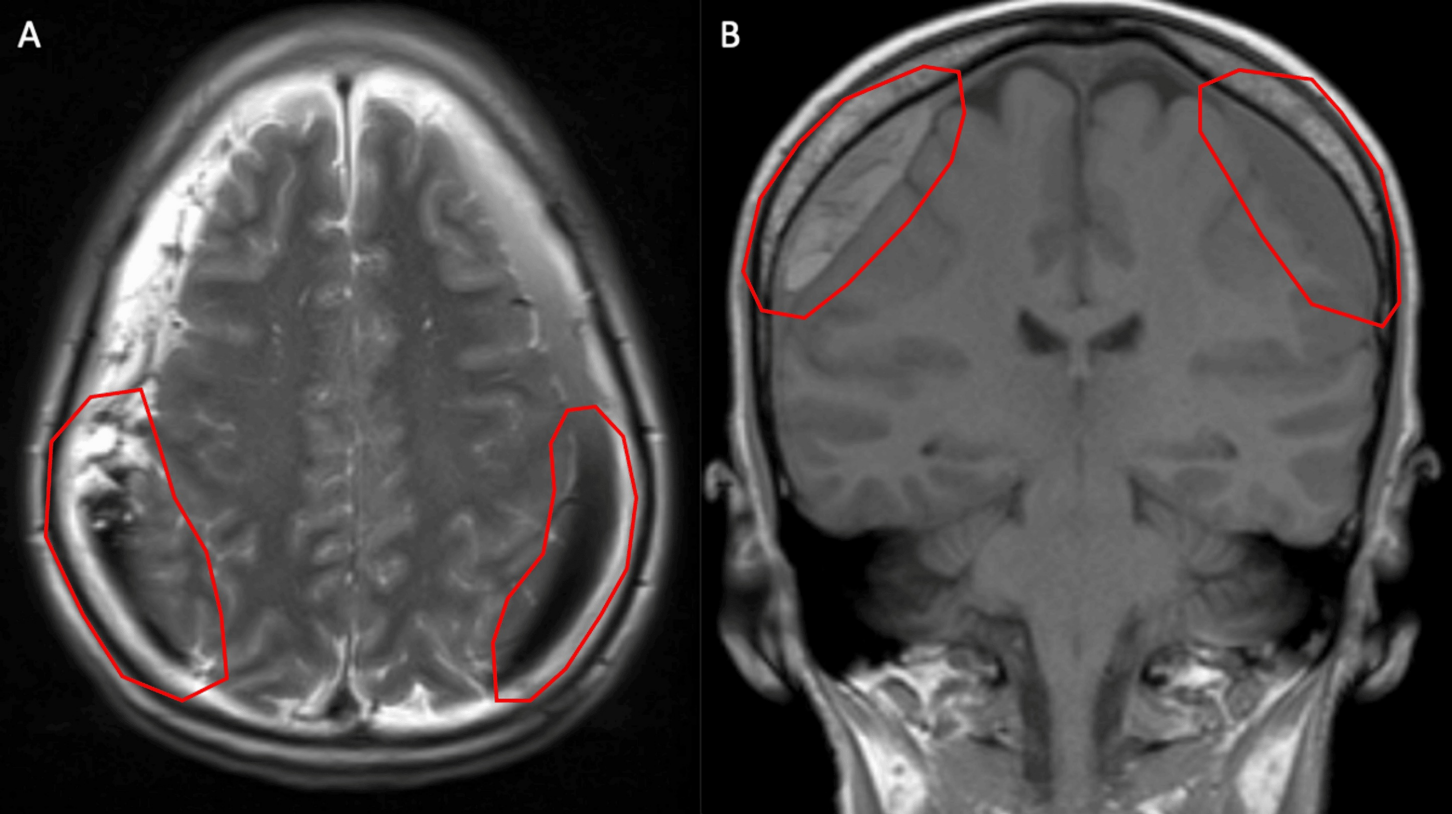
Axial and coronal MRIs of subdural hematomas A) Axial MRI demonstrating bilateral subdural hematomas with mild localized mass effect upon the cerebral hemispheres (red). B) Coronal MRI demonstrating bilateral subdural hematomas with mild localized mass effect upon the cerebral hemispheres (red).
